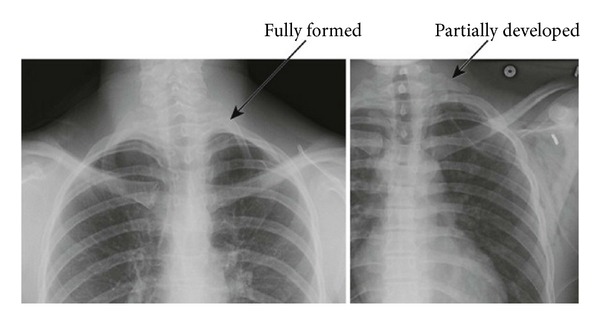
Radiographs show both a fully formed and a partially developed cervical rib (reprinted with permission of Elsevier; see [8]).
